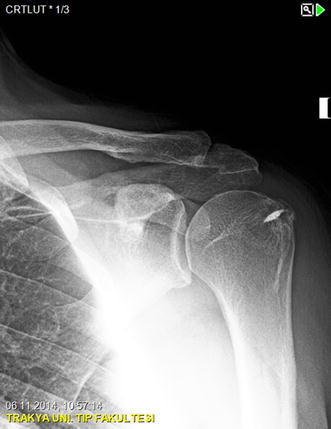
Follow-up roentgenogram, postoperative 2 years
